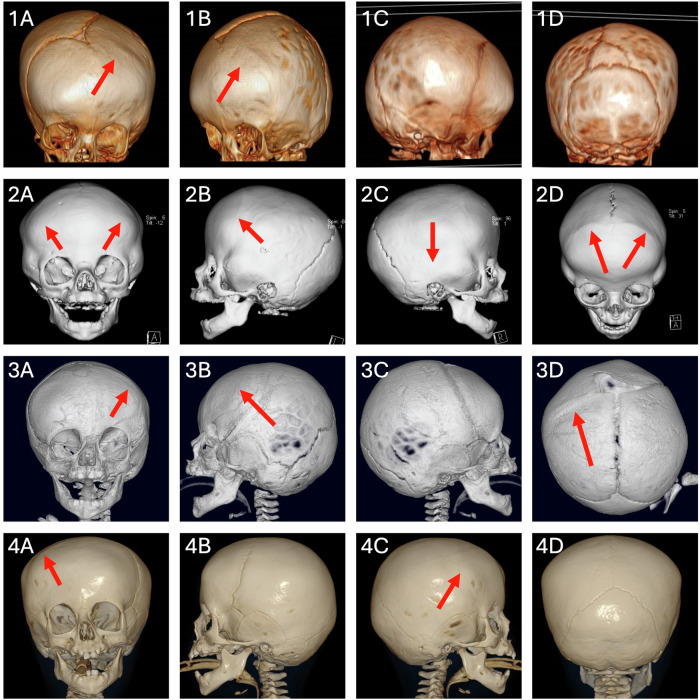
Pre-operative images of 3D CT scans of Patients 1-4. Patient 1 (1A–1D), Patient 2 (2A–2D), Patient 3 (3A–3D) and Patient 4 (4A–4D). The red arrows indicate prematurely fused sutures.
